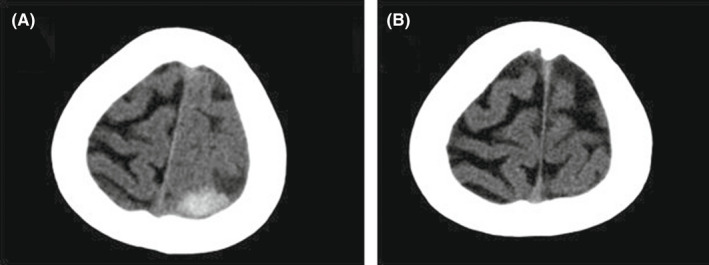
(A) Spiral brain computed tomography (CT) showed evidence of acute and subacute foci of For Review Only subdural hematoma adjacent to the left temporal lobe. (B) The hemorrhagic lesion disappeared after 1 month
